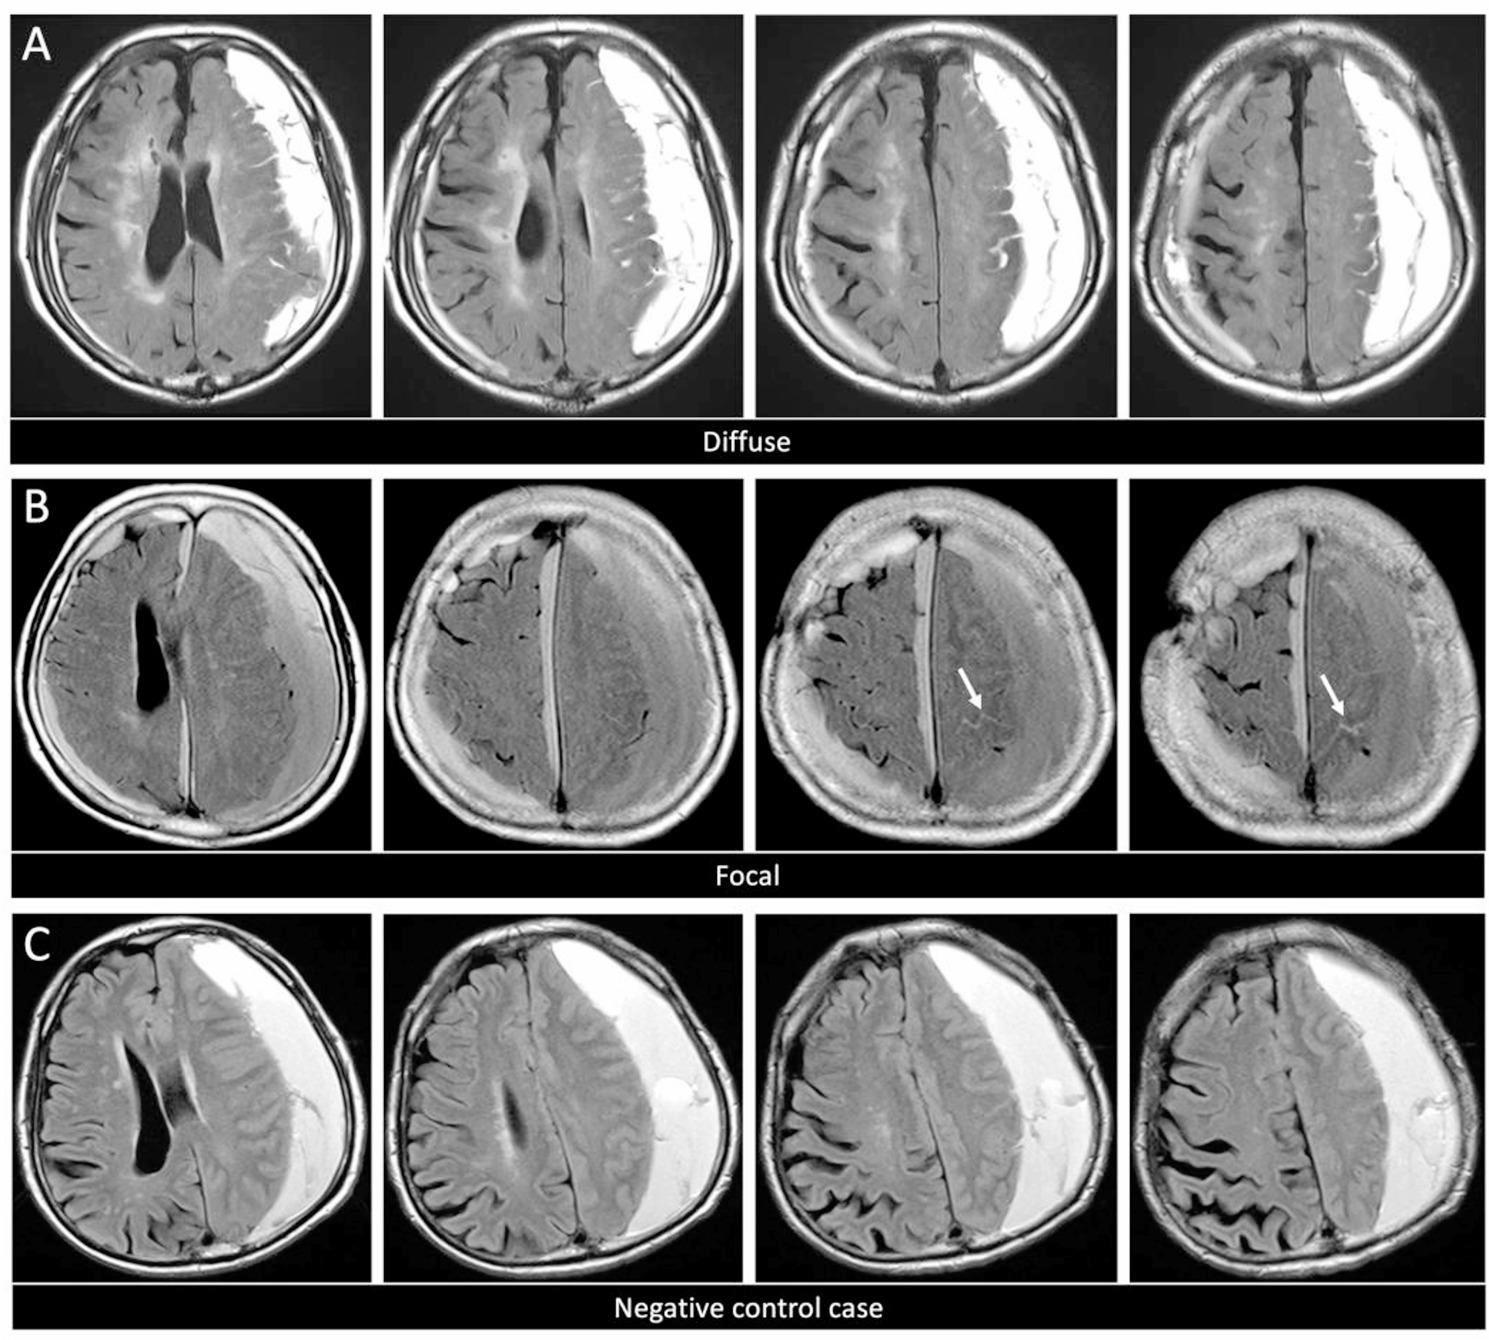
Sulcal hyperintensity on FLAIR in a patient with chronic subdural hematoma. A Diffuse sulcal hyperintensity (involving two lobes). B Focal sulcal hyperintensity (confined to a single lobe, white arrow). C Negative control case. Abbreviations: FLAIR; fluid attenuated inversion recovery
